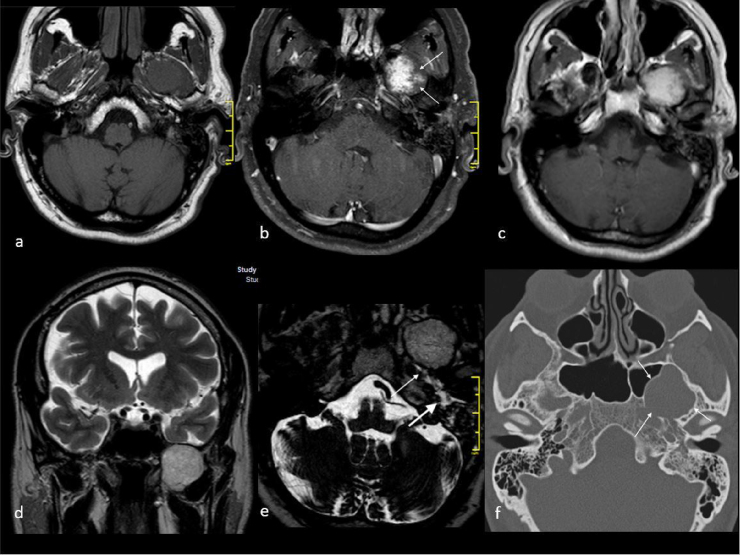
(a) Ax T1 image shows homogeneous iso-signal of the lesion to muscle. Early post gd injection on B image (ax T1 3D fat sup) shows incomplete enhancement (arrow). A few minutes after gd injection on c image (Ax T1 se) the enhancement is complete, homogeneous. On T2 wi (d image, coronal turbo T2) the lesion shows high homogenous signal. On Ax T2 3D image (e) it’s clearly evident the high signal of retention material in middle ear and in the tuba (arrows). CT bone image (f), the bone is remodeled by lesion expansion (arrows) without erosion, typical aspect for long standing lesion.
